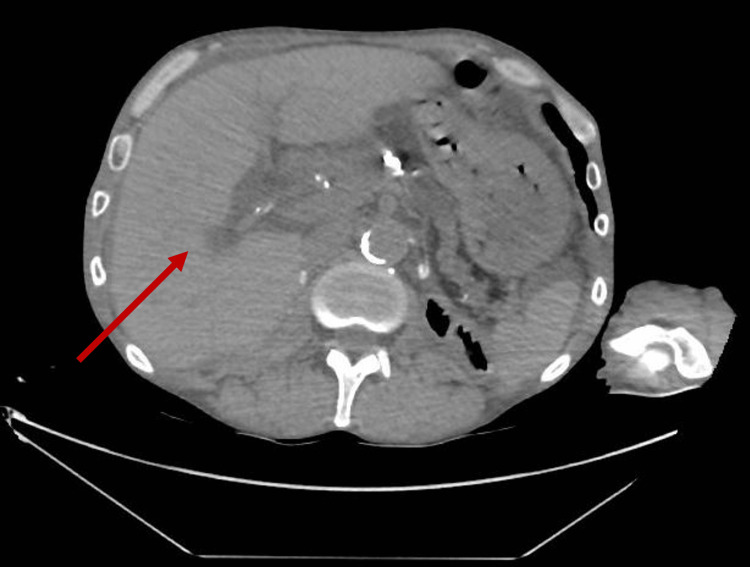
CT Abdomen/Pelvis (axial view) The image is showing resolution of the previous HPVG (Hepatic Portal Venous Gas)
